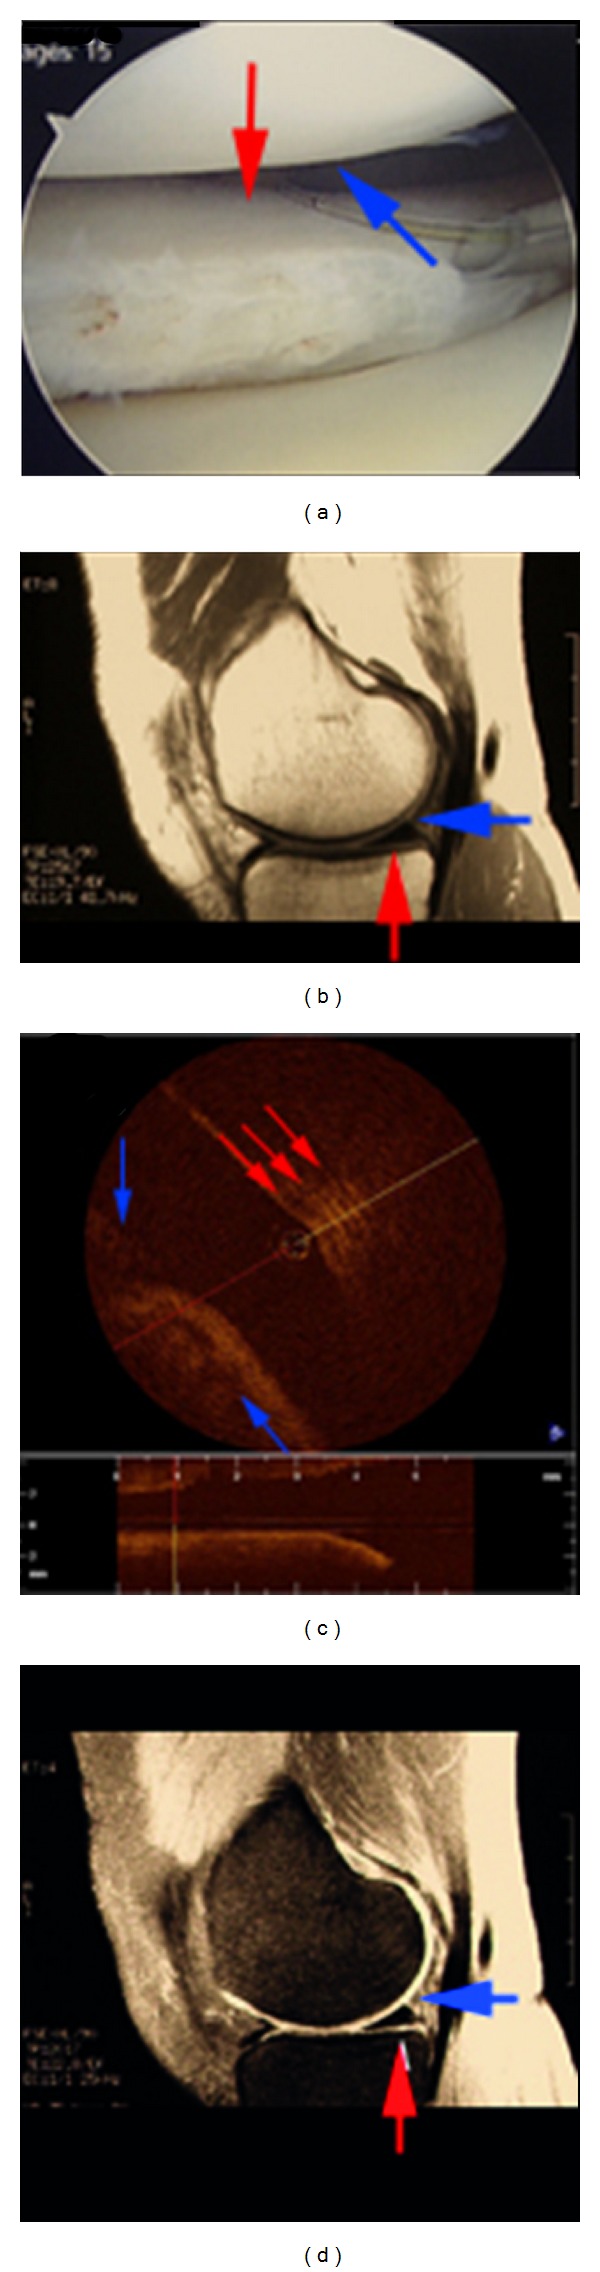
(a) Arthroscopic image of normal meniscal cartilage and mildly diseased femoral cartilage. The red arrow represents a section of meniscus, and the blue arrow represents a section of mildly diseased cartilage. Both of these positions look normal from the arthroscopic image. (b) MR image of the joint using a T1 and T2 (top to bottom) relaxation states. The sagittal cross-section shows a heterogeneous articular layer at the blue arrow and a normal meniscus at the red arrow. (c) OCT image of area represented by the arthroscopic image. The OCT image shows a relative loss of a uniformed banding pattern at the blue arrow indicative of collagen breakdown in the cartilage yet shows a tight uniformed banding pattern at the red arrow indicative of healthy highly organized meniscus. Image courtesy of Zheng et al. [9].
